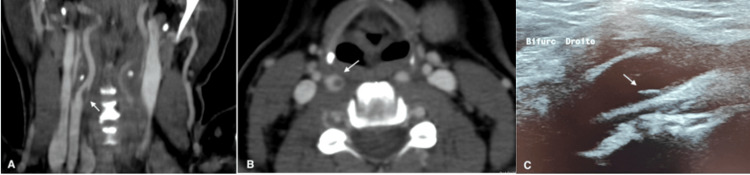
(A) Cross-sectional computed tomography angiography (CTA) image of Patient 3, revealing a carotid web accompanied by a free-floating thrombus within the internal carotid artery lumen. (B) Axial CTA view highlighting the characteristic “donut sign” . (C) Duplex ultrasound image obtained post-thrombus resolution, clearly delineating the prominent carotid web projecting into the arterial lumen.
